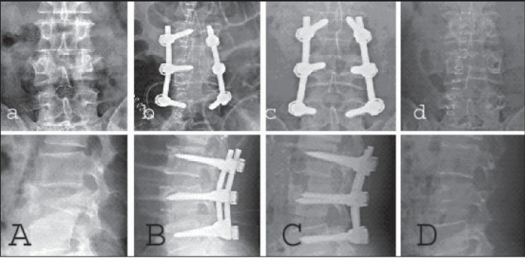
AP & Lateral x-ray showing L_3_ burst fracture: Preoperative (A) and postoperative at 0 (B), 12 months (C), 13 months after implant removal (D)
